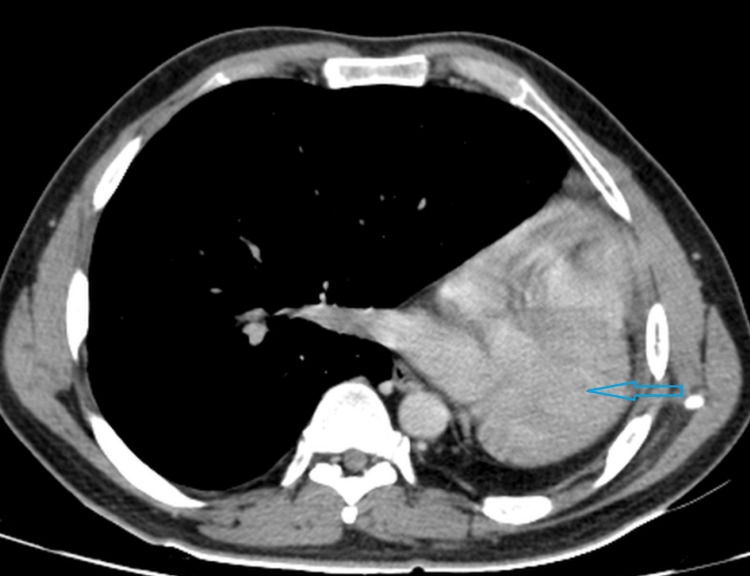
Contrast CT scan of the chest in mediastinal window shows the heart displaced in the left hemithorax along the chest wall CT: computed tomography
